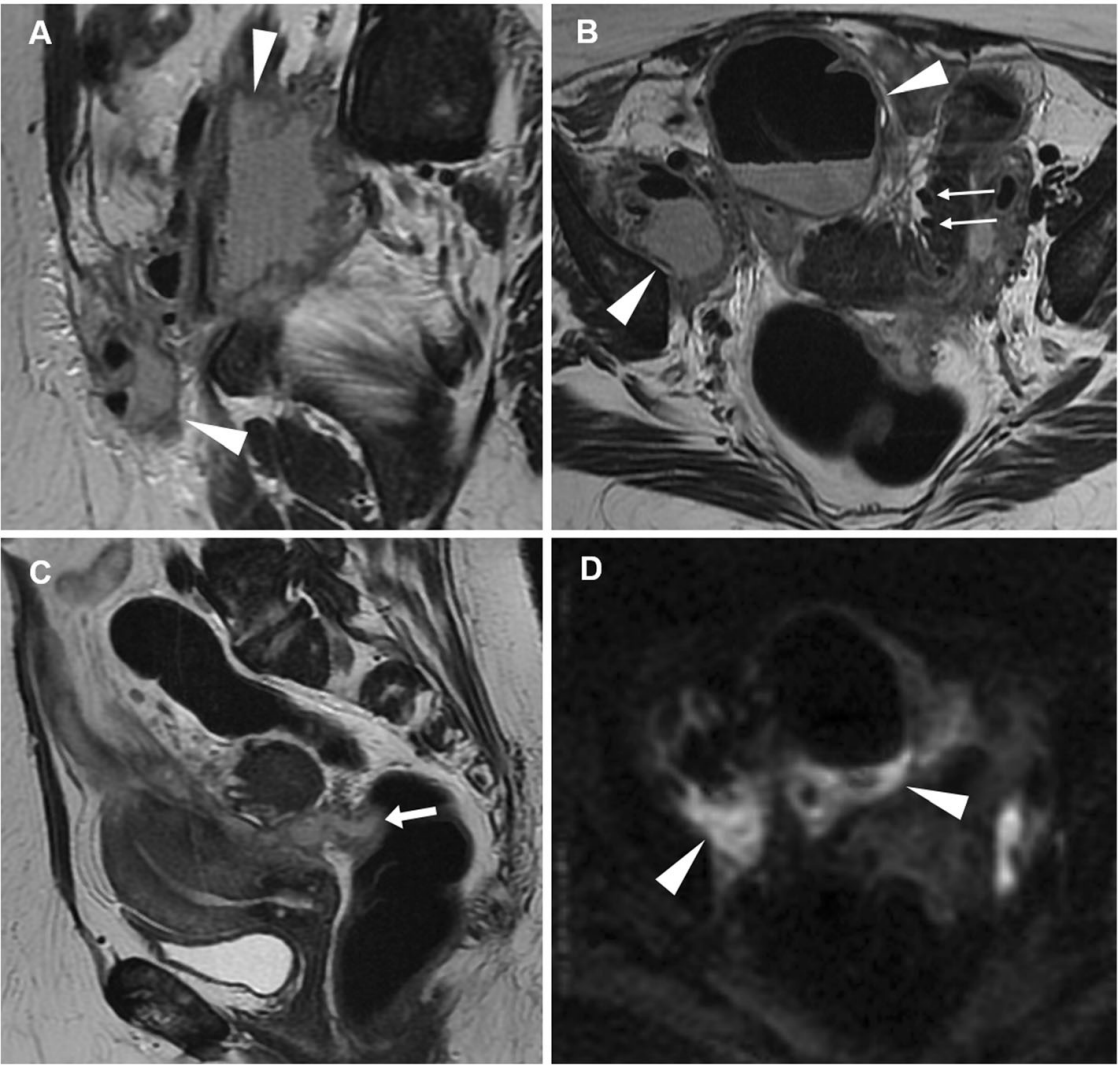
TOA in a 57-year-old woman with right lower quadrant pain, fever, leukocytosis and acute diverticulitis. Sagittal (a) and axial (b) T2-weighted images show heterogeneous, multilocular collections at the right adnexa (arrowheads), containing air-fluid levels. Note diverticula of the sigmoid colon (thin arrows in b). Sagittal T2-weighted image (c) displays a fluid collection in the Douglas pouch (arrow). On axial DWI (*b* = 800 s/mm^2^) image (d), the fluid component of the adnexal masses demonstrates high signal intensity (arrowheads), consistent with restricted diffusion from purulent content
